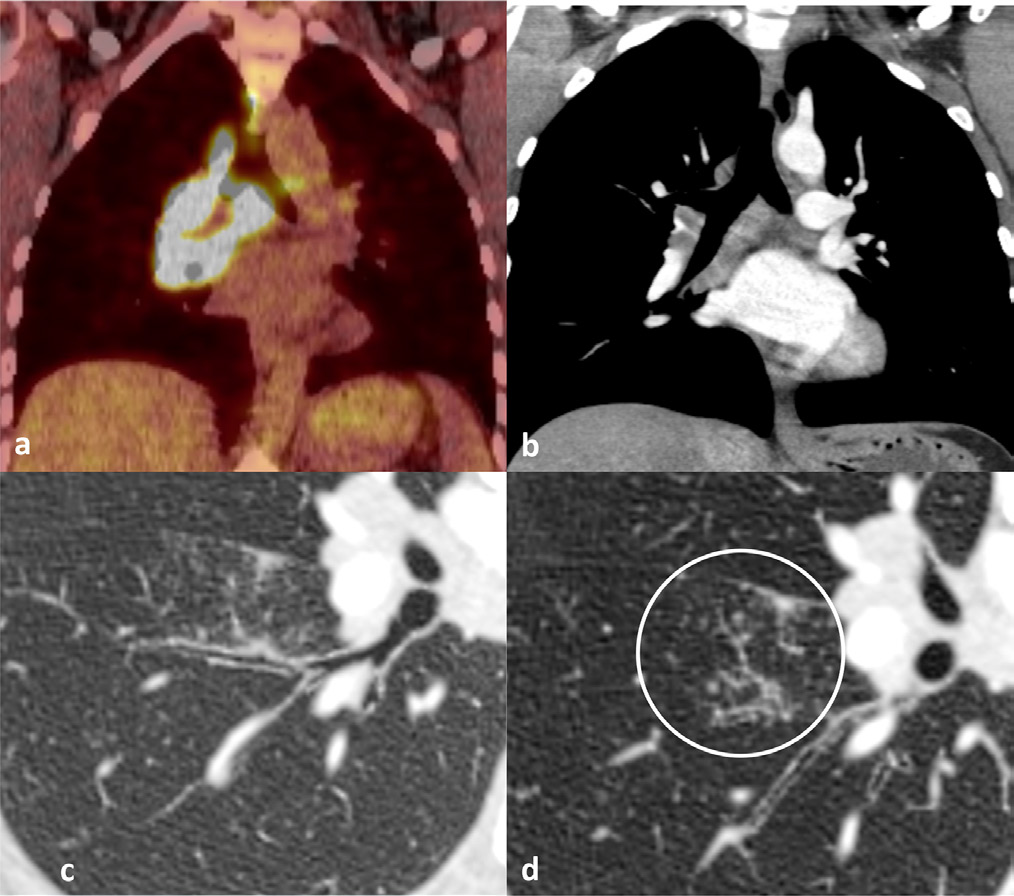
CoronalFDG-PET/CT (a) and equivalent post contrast coronalreformatted CT (b) showing right paratracheal, hilar and subcarinal lympadenopathy. The HRCT component of the CT shows asubtle focal area of thickening of the bronchovascular tree and oblique fissure (c) and tinynodules (circle) in the right lower lobe (d).
